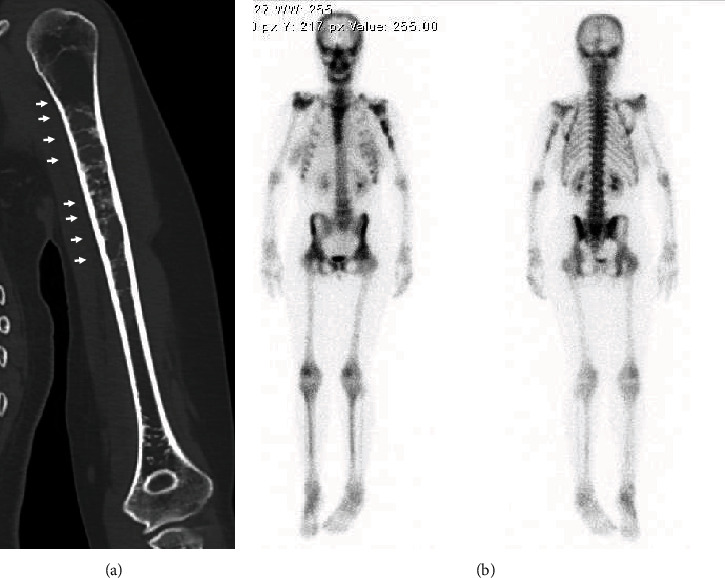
Plain computed tomography and bone scintigraphy. (a) Plain computed tomography showing calcification in the diaphysis of the left humerus (arrows). (b) Bone scintigraphy demonstrating increased focal uptake in the lesion (arrows).
